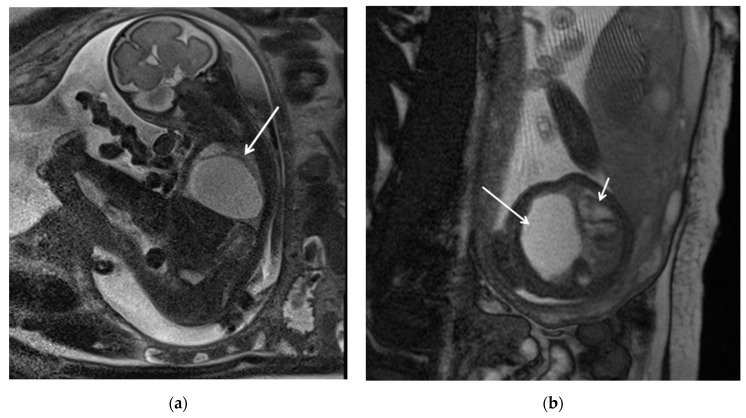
CPAM type I. HASTE oblique sagittal (a) and True FISP (b) MRI scans taken at 28 weeks of gestation reveal a large fluid-filled mass with bright T2 signal in the right chest (arrows). This abnormality results in a shift of the heart toward the left side of the mediastinum.
